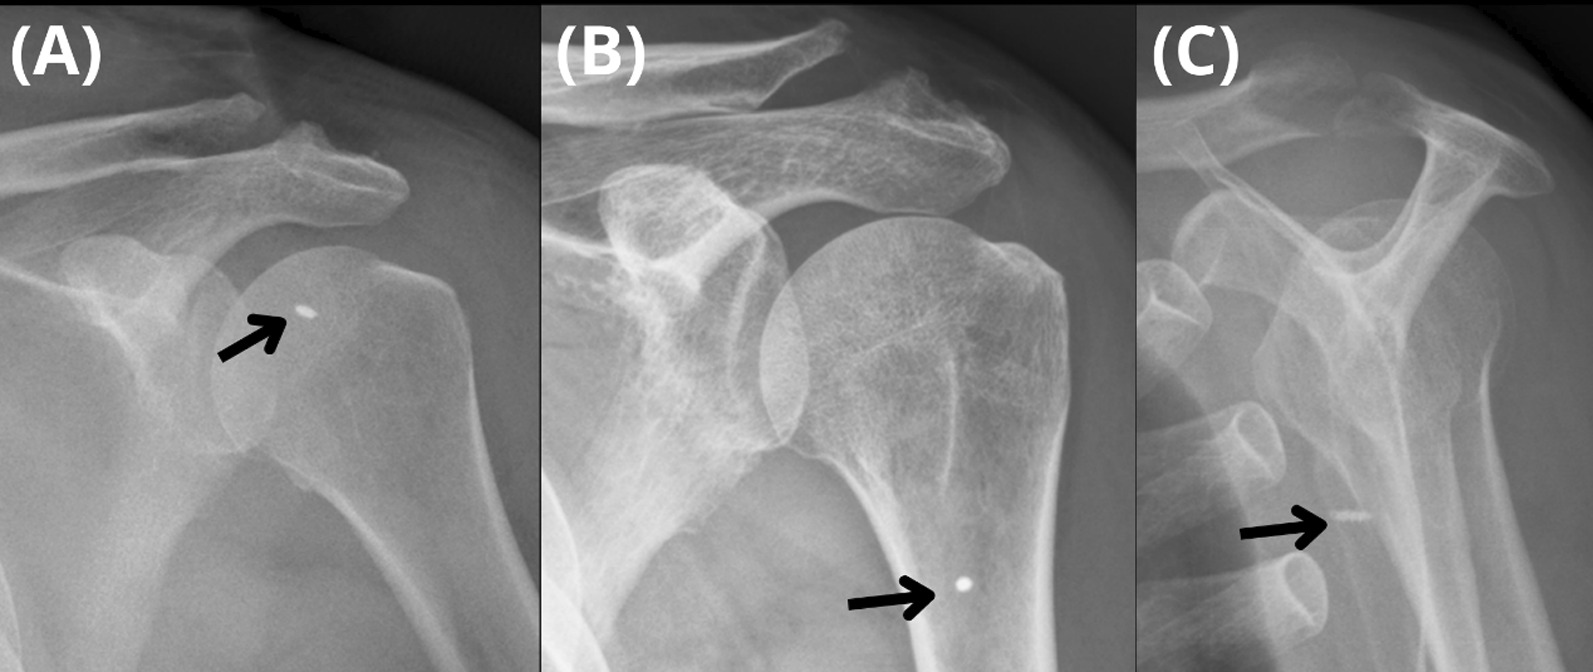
A A metallic anchor (arrow) is fixed at the proximal inlet of the bicipital groove. One month after the index surgery, the metallic-anchor pullout is observed on B standard anteroposterior and C shoulder outlet radiographs
